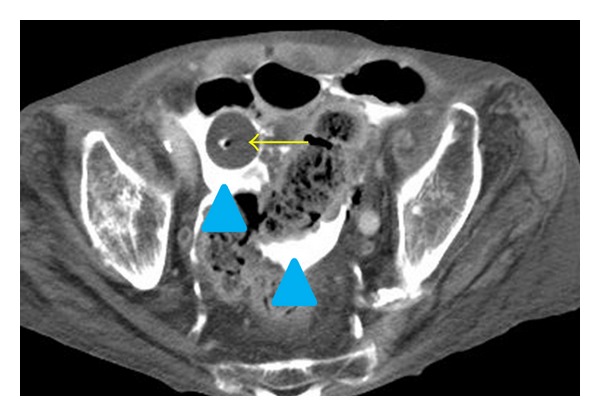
Contrast-enhanced computed tomography after transurethral perfusion of contrast medium demonstrated extravasation of the contrast material into the peritoneal cavity (▲). In addition, the Foley catheter balloon (arrowhead) was located within the peritoneal cavity through the urinary bladder.
